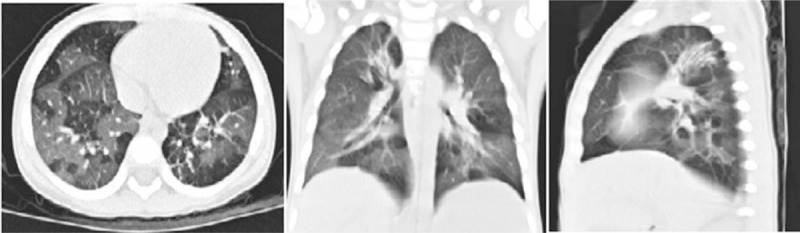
HRCT image showing bilateral mosaic ground-glass patterns with air trapping.
